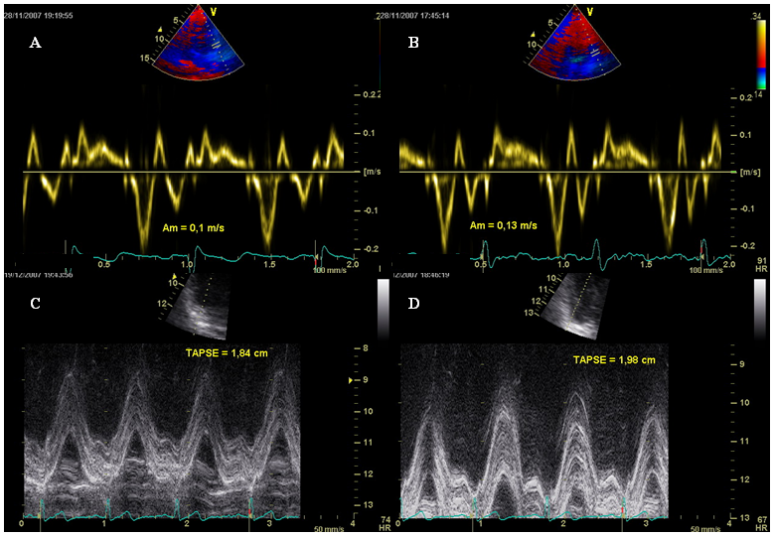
Tissue Doppler pattern of mitral annulus motion and M-mode imaging of tricuspid annulus motion at baseline (panels A-C) and after cigarette smoking (panels B-D), showing an increase in mitral peak A_m _velocity and tricuspid annular plane systolic displacement (TAPSE).
